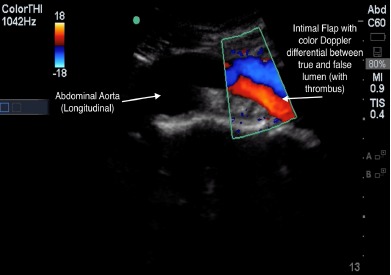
Longitudinal view of abdominal aortic dissection with true lumen, intimal flap, and echogenic false lumen. Superimposed color Doppler demonstrating flow differences in true and false lumen of abdominal aortic dissection.
